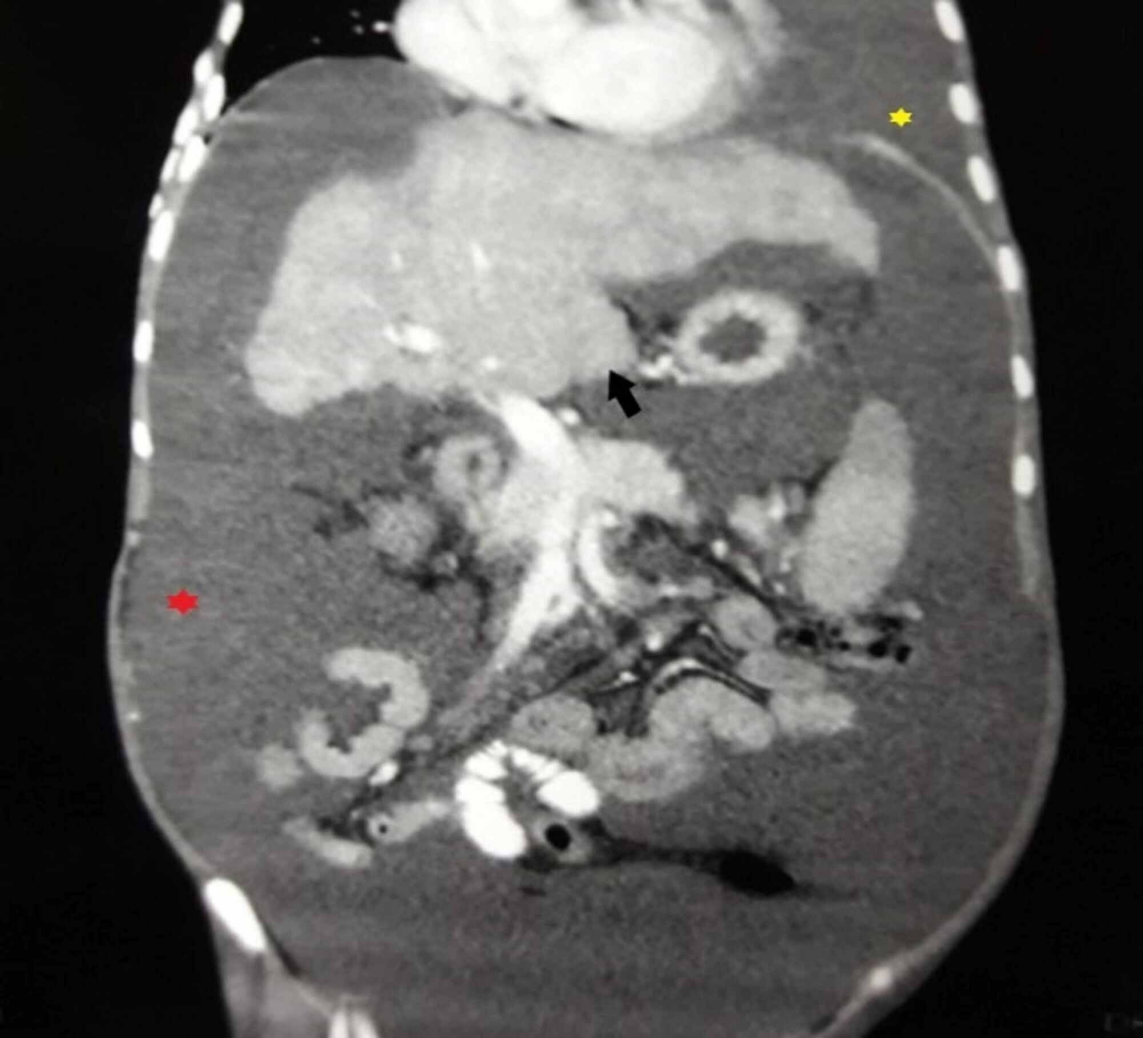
Coronal contrast computed tomography (CT) obtained during venous portal phase shows altered liver parenchyma with hypertrophy of caudate lobe (black arrow). Left pleural effusion (yellow *) and massive ascites (red *) can also be seen.
